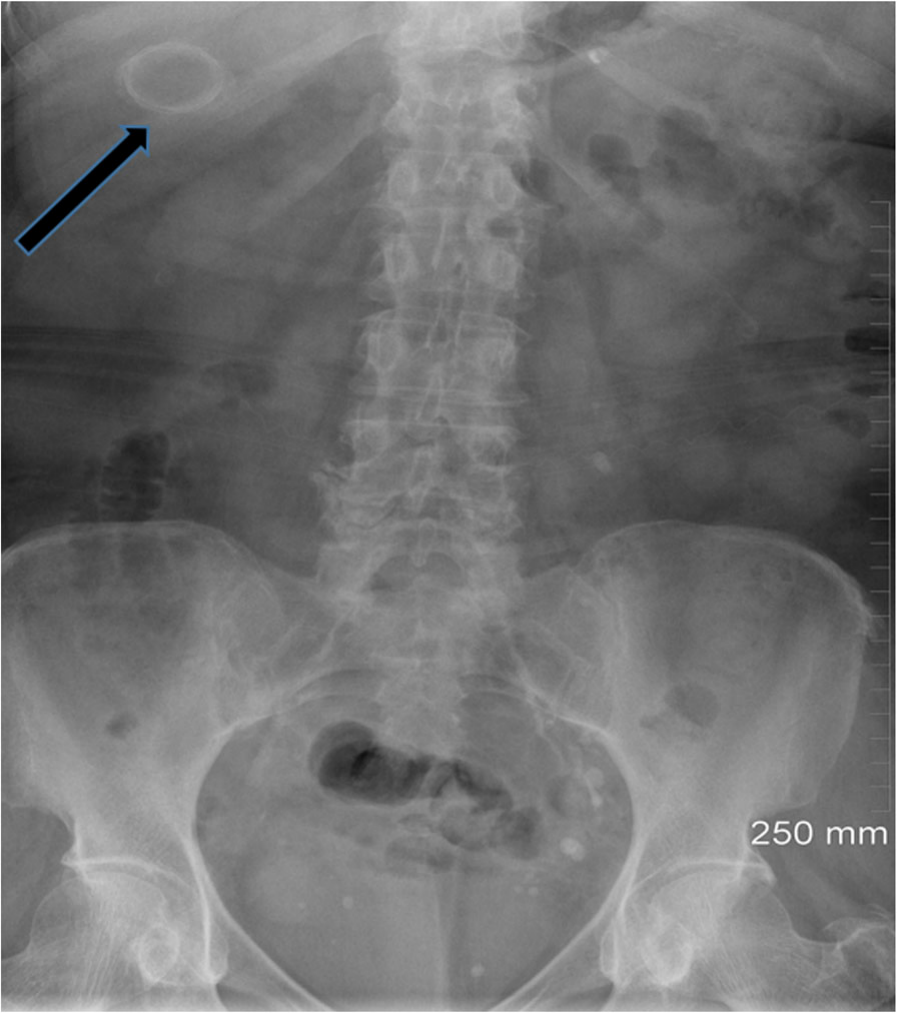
Abdominal radiograph revealing a large rounded calcified density with a central lucency in the right upper quadrant consistent with a peripherally calcified gallstone
